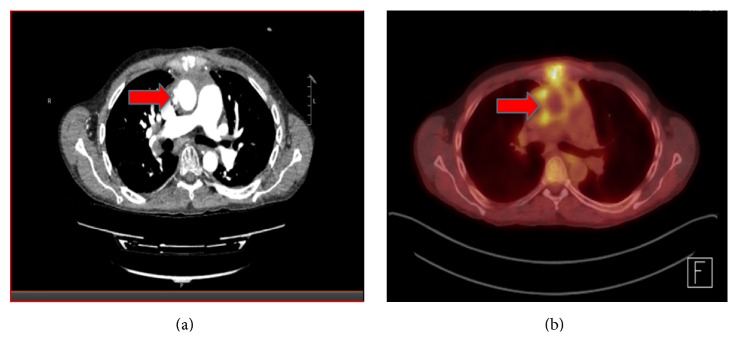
Cross-sectional views of CT angiogram image (a) and PET image (b) of the patient's chest, showing two outpouchings in the wall of ascending aorta (pointed by red arrows) which were FDG avid on the PET, indicative of mycotic pseudoaneurysms.
